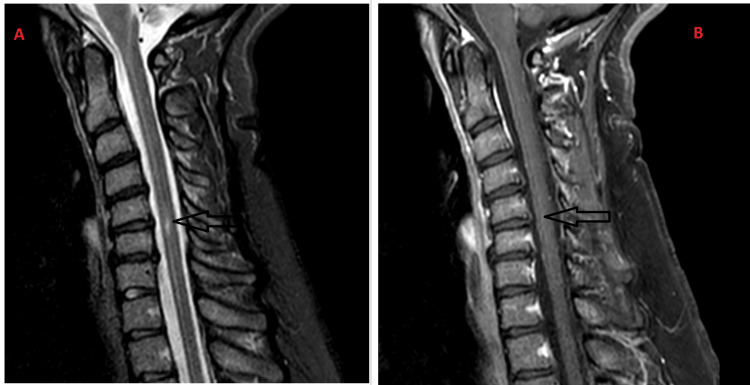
C-spine MRI (On Follow-up) 2A. Sagittal C-spine MRI with a focus of increased T2/STIR signal between C5-C6. Degenerative changes and disc bulges involving C4-C7, rectification of cervical cord, and improved reversal of cervical lordosis. 2B. Postcontrast T1 sagittal images with no abnormal enhancement within the cervical cord. C-spine: cervical-spine; STIR: short tau inversion recovery
